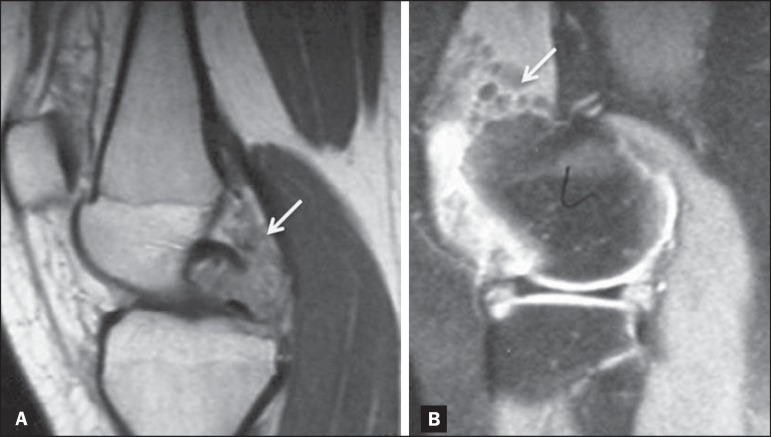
Lipoma arborescens. PD-weighted MRI sequences, both in the sagittal
